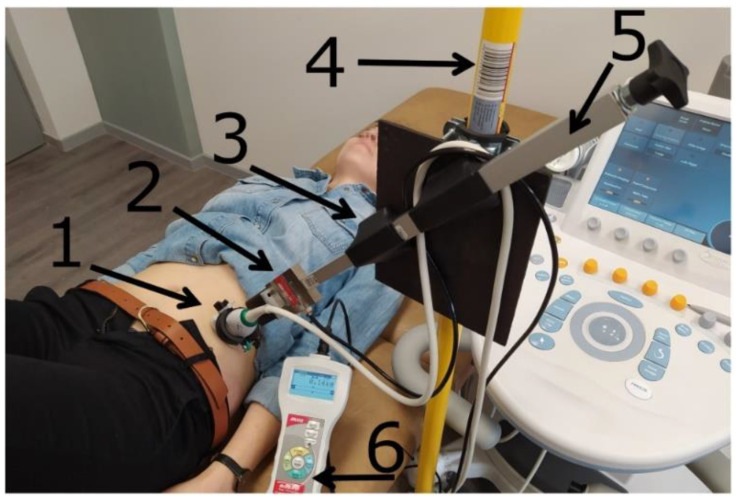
General overview of the setup for the ultrasound examination with probe compression controlled. 1. Ultrasound probe. 2. External S-Type load cell connected to a force gauge (6). 3. Two-dimensional magnetic holder. 4. An expansion beam. 5. Precise screw actuator.
